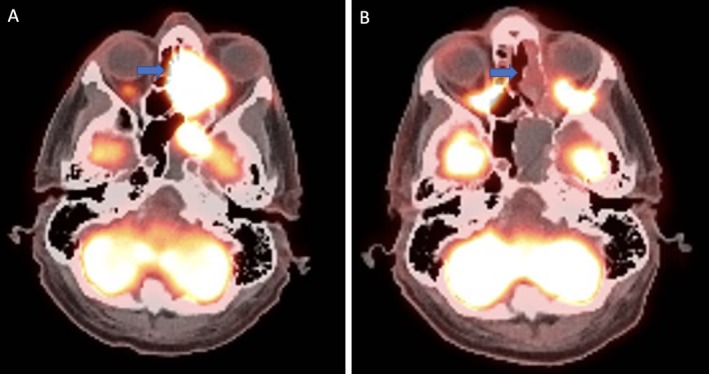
(A) Baseline FDG‐PET imaging demonstrating large hypermetabolic lesion in left nasal cavity. (B) FDG‐PET showing no FDG‐avid lesions in the nasal cavity after cycle 7.
